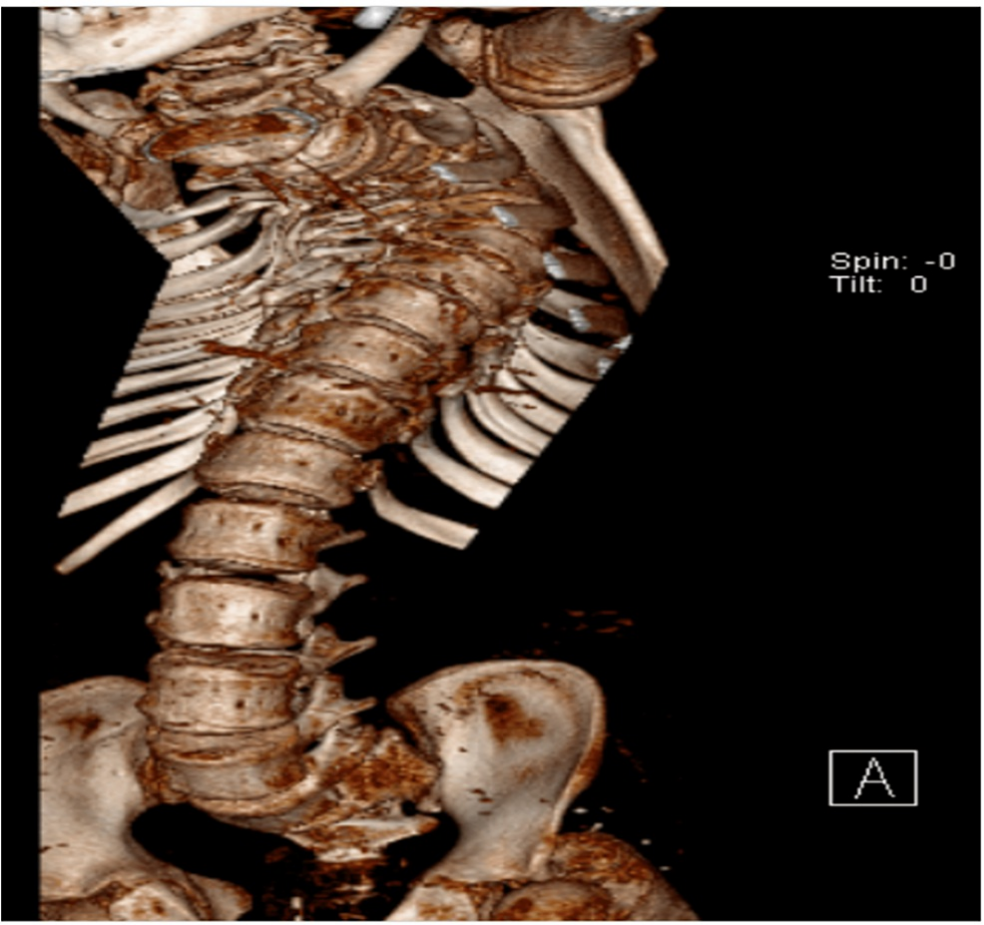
2D CT showed significant spinal kyphoscoliosis deformity
